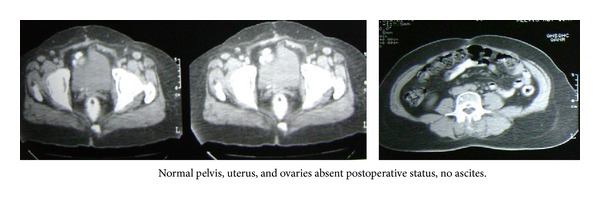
CT scan after 24 weeks following 6 cycles.
